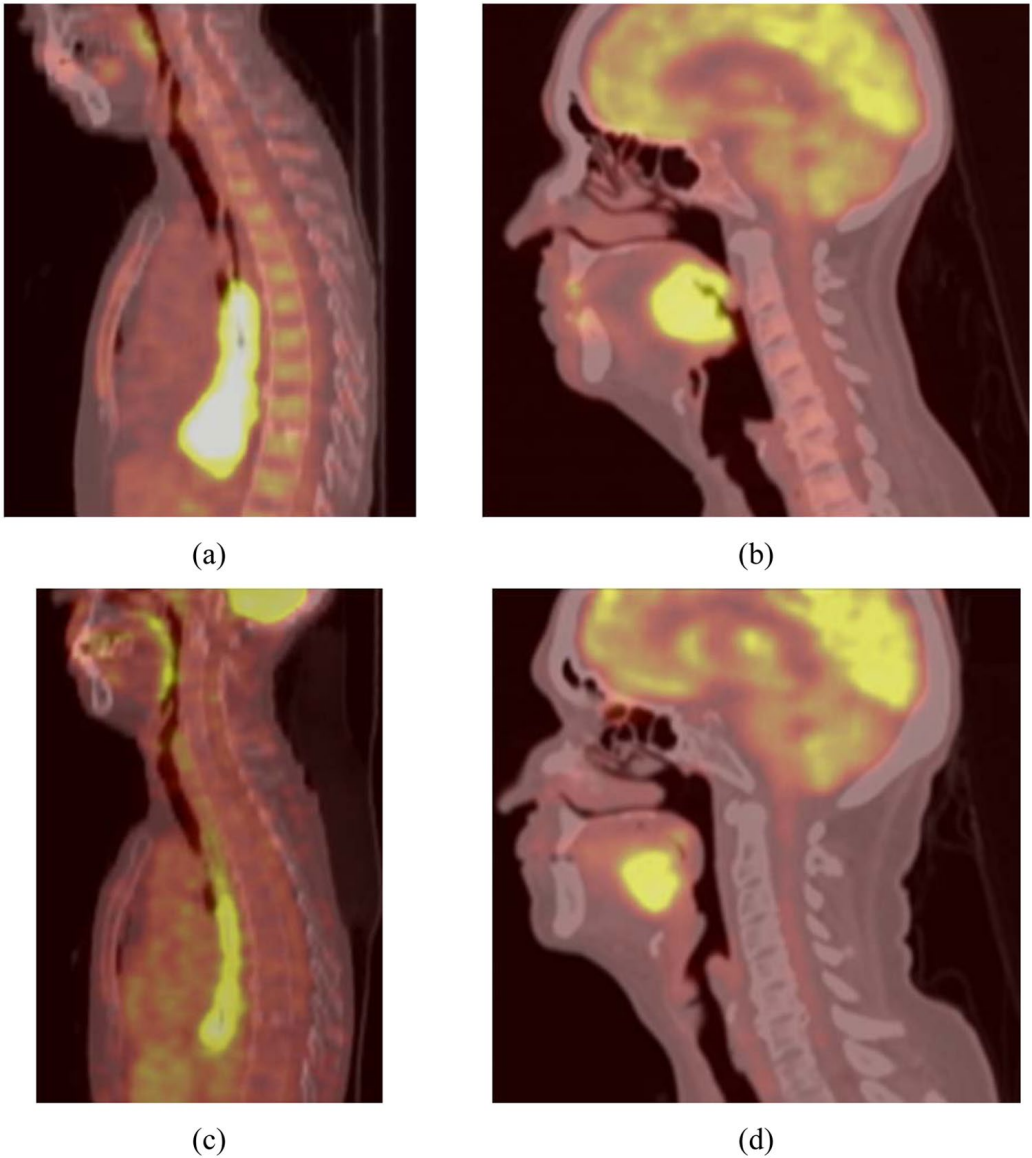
Examples of (a,c) pre-treatment and (b,d) post-treatment PET/CT images in (a,b) esophageal and (c,d) H&N tumors.
